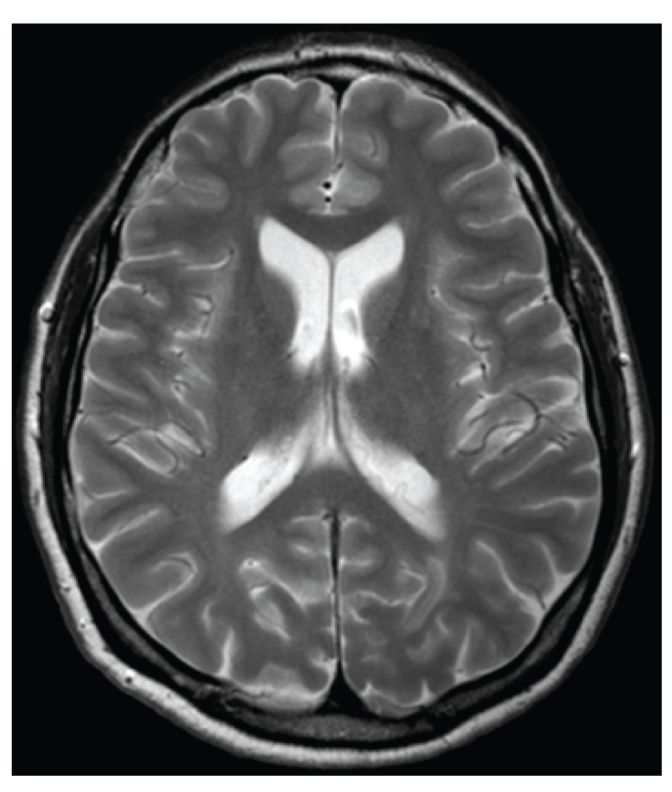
Axial T2-weighted brain MRI 2 months later shows complete resolution of the occipital lesion.
